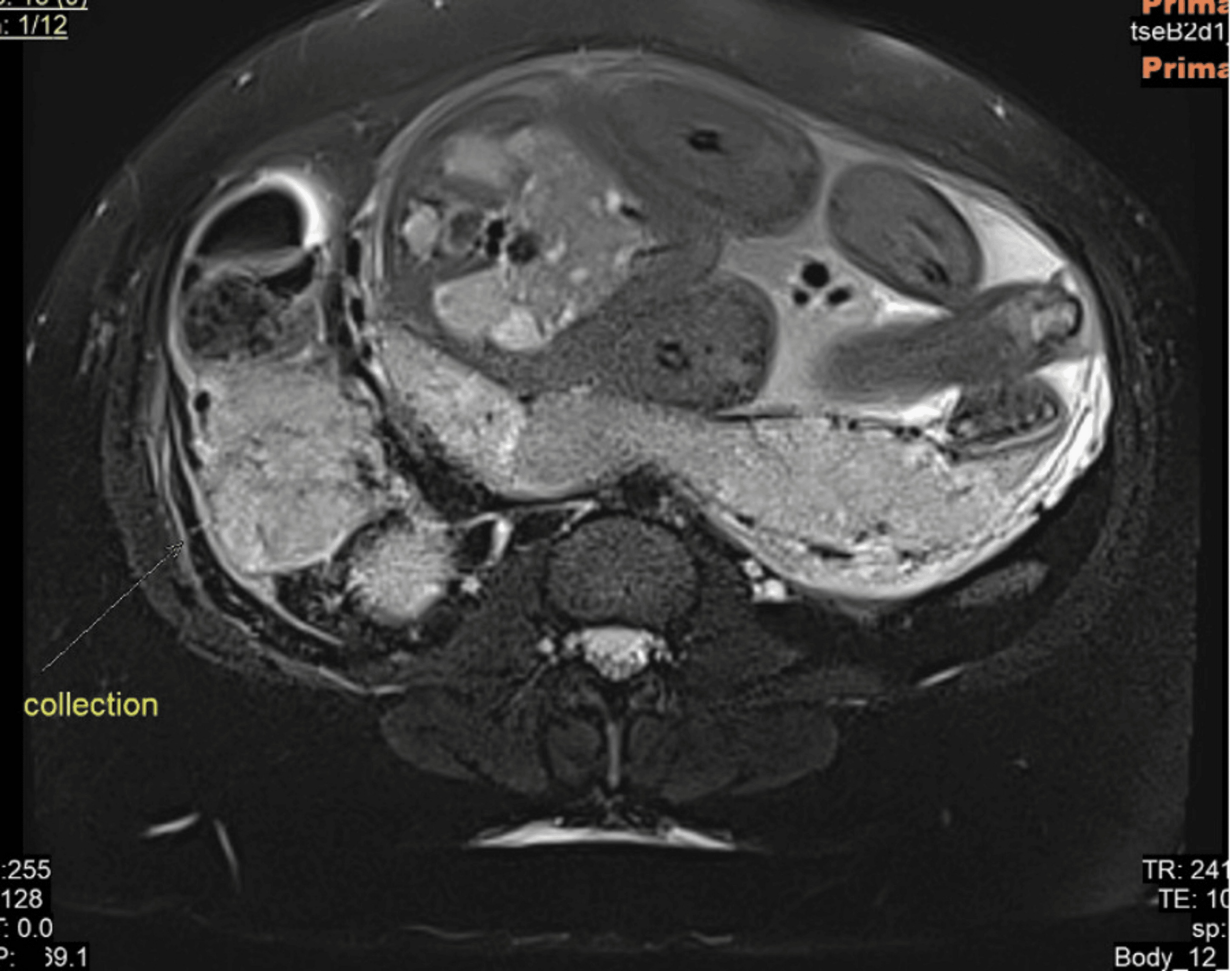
Magnetic resonance imaging of the abdomen. Magnetic resonance imaging of the abdomen revealing the subhepatically located appendix that was dilated to approximately 1 cm and associated with a large subhepatic phlegmon measuring 5.7 × 4.2 cm, along with small, tiny non-drainable abscess pockets.
